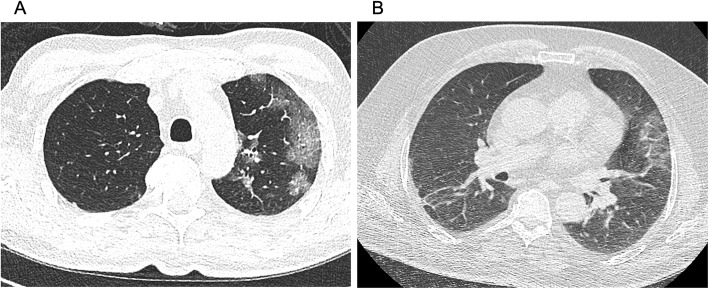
Two COVID-19 patients with initially negative RT-PCR, but highly suspicious findings in initial CT scan. Patient A was tested positive for COVID-19 in the second RT-PCR and patient B in the fourth, while initial CT scan already revealed typical findings
